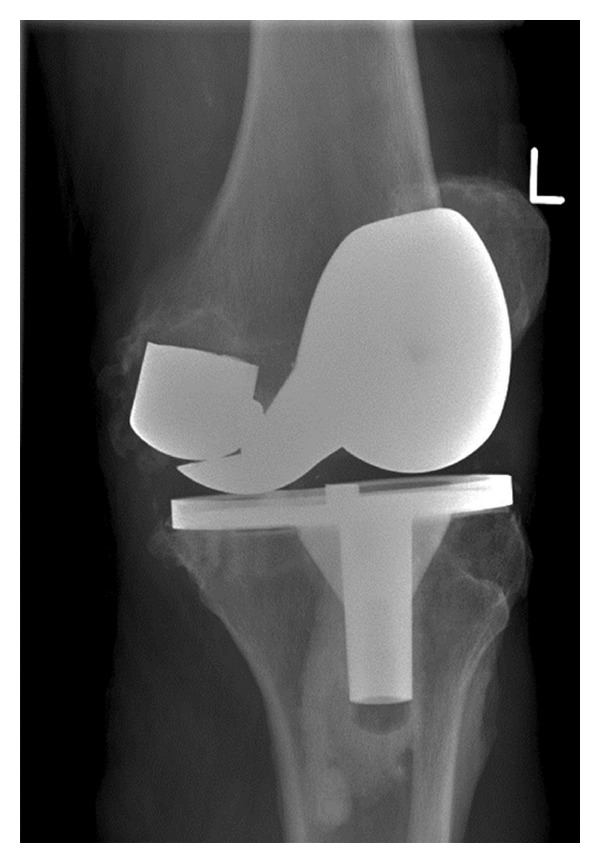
An oblique radiograph of the knee demonstrating the broken prosthesis.
